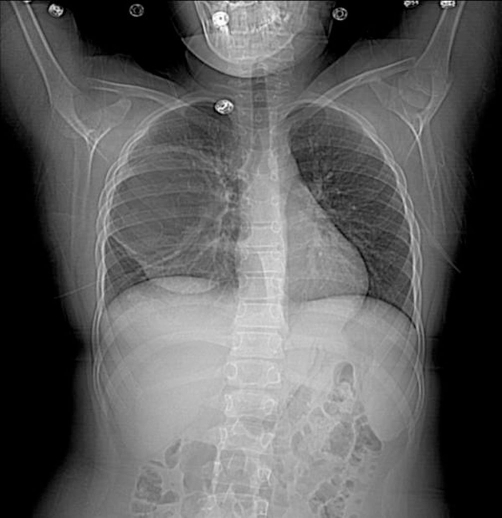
Initial chest X-ray revealing bronchogenic cyst in the posterior right middle lobe (10 × 10 × 8 cm^3^).
